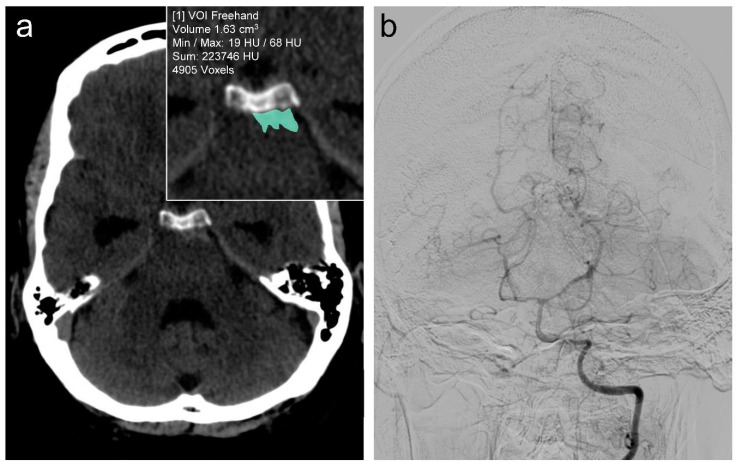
Exemplary patient case with pmSAH (modified Fisher scale 3) and the corresponding segmentation (green) in the magnification (a). The complications of hydrocephalus can be seen by the dilated temporal horns on non-contrast CT and the vasospasms in the posterior circulation (diagnostic angiogram via left vertebral artery indicating the segmental narrowing of basilar artery and posterior cerebral arteries on both sides, (b)).
